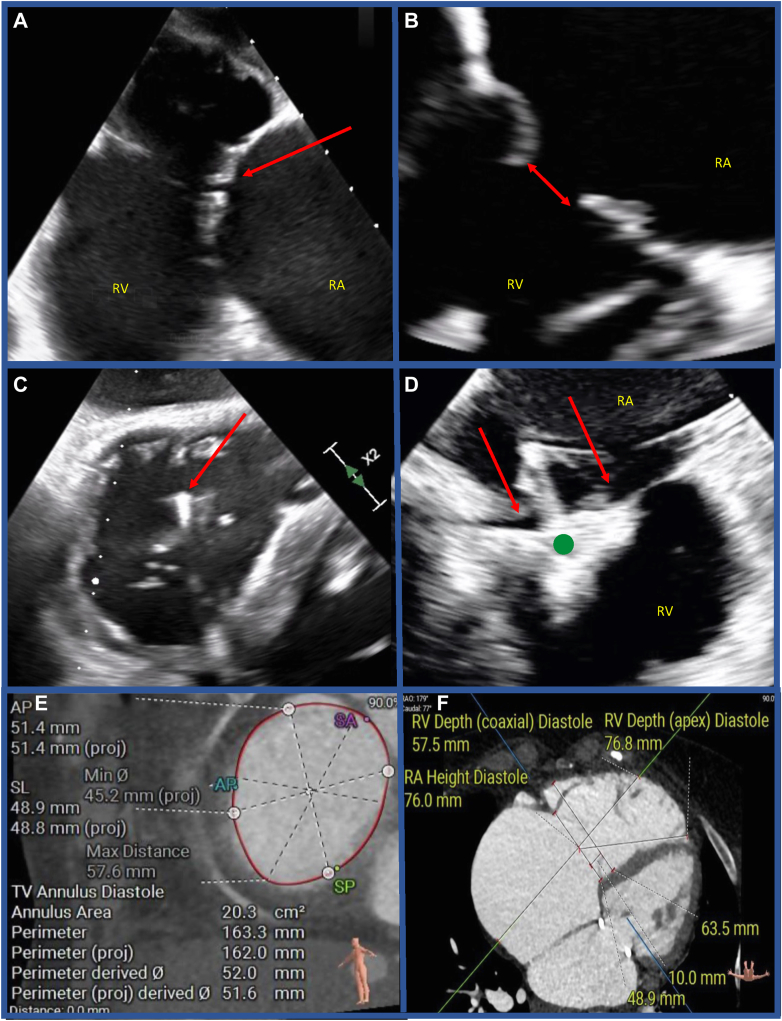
Imaging of the tricuspid valve in TTVI. (a) Transesophageal images showing adequate image quality with desirable anatomical features for T-TEER with good leaflet length, mobile leaflets, and a reasonable coaptation gap (red arrow). (b) Transesophageal echocardiographic images showing suboptimal anatomical features for tricuspid T-TEER, with a wide coaptation gap (red arrow). This patient was referred for TTVR. (c) Transesophageal echocardiographic images showing suboptimal image caused by artefact from a transvalvular pacing lead (red arrow). This patient underwent T-TEER supported by ICE. (d) Using ICE, good quality images of the clip (green dot) and valve leaflets (red arrows) were obtained during T-TEER, despite the presence of pacing leads, in the patient from Panel C. (e) CT annular dimensions in the diastolic phase, showing the anteroposterior and septolateral dimensions, as well as the annular perimeter. (f) CT 4-chamber view obtained during work-up for TTVI in a patient who was anatomically unsuitable for T-TEER. This section demonstrates the RV and RA heights, which in addition to annular dimensions comprise the CT screening parameters for TTVR Abbreviations: AP, anteroposterior; ICE, intracardiac echocardiography; RA, right atrium; RV, right ventricle; SA, septoanterior; SL, septo-lateral; SP, septoposterior; T-TEER, transcatheter tricuspid valve edge-to-edge repair; TV, tricuspid valve; TTVI, transcatheter TV intervention.
